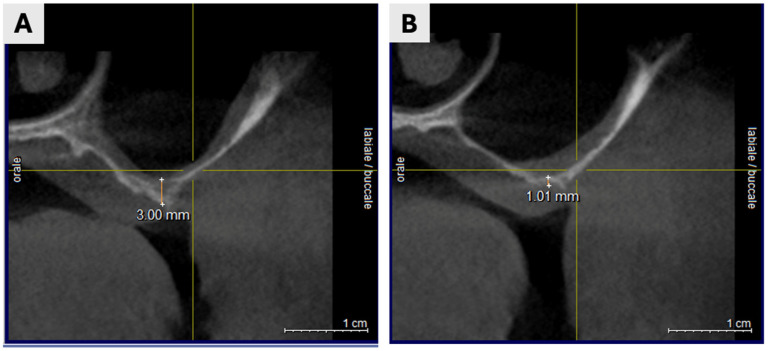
Pre-operative cone-beam computed tomography (CBCT) assessment of residual bone height in the posterior maxilla. (A) Right maxillary sinus showing a residual bone height of 3.00 mm. (B) Left maxillary sinus showing a residual bone height of 1.01 mm. No sinus pathology, septa, or Schneiderian membrane abnormalities were detected.
